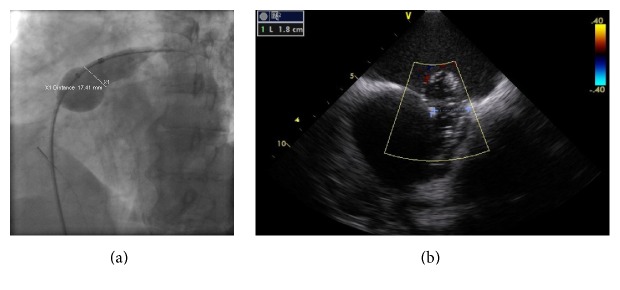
(a) Resizing of the ASD using the sizing balloon. (b) Resizing of the ASD using the intracardiac echocardiogram.
